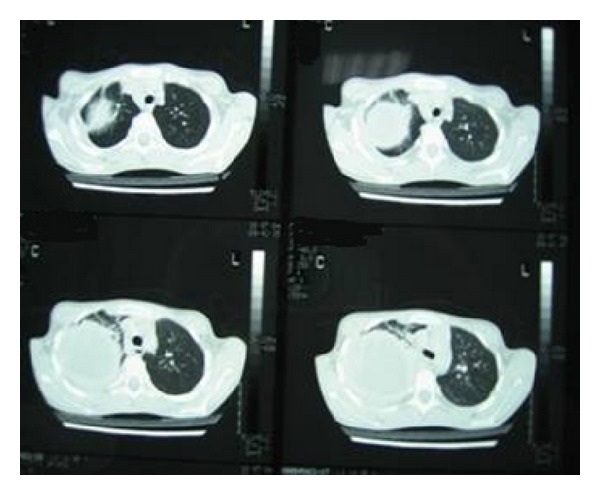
Computed tomography showed a large space-occupying solid and homogeneous right thoracic/mediastinal mass associated with pleural effusion.
